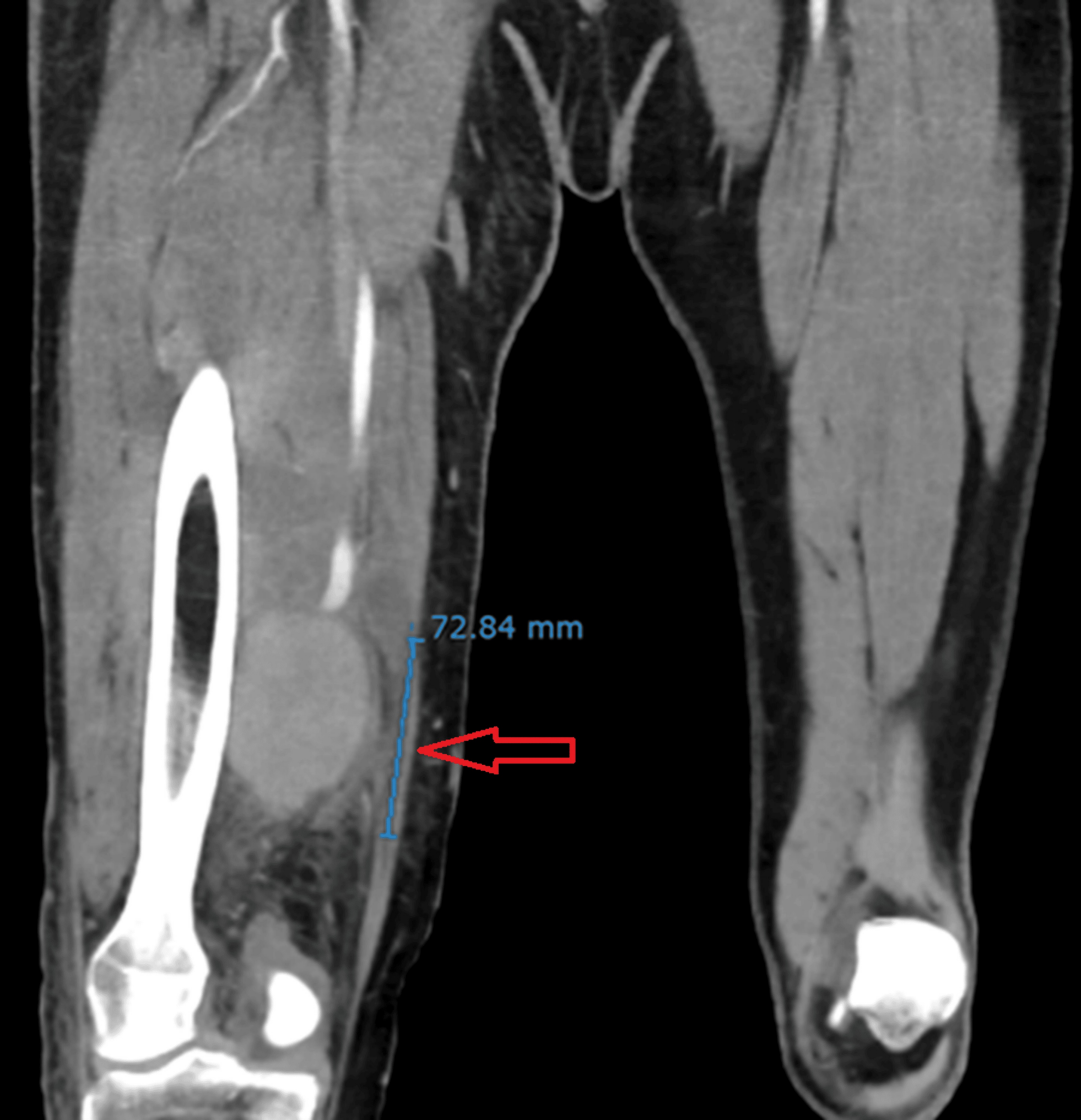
Coronal CT angiogram of right superficial femoral artery aneurysm Contrast-enhanced coronal CT angiography demonstrates a large, saccular aneurysm (red arrow) arising from the right superficial femoral artery, measuring approximately 6.4 × 6.3 × 7.3 cm in cross-section. There is subcutaneous soft tissue stranding in the posterior right thigh and popliteal fossa.
